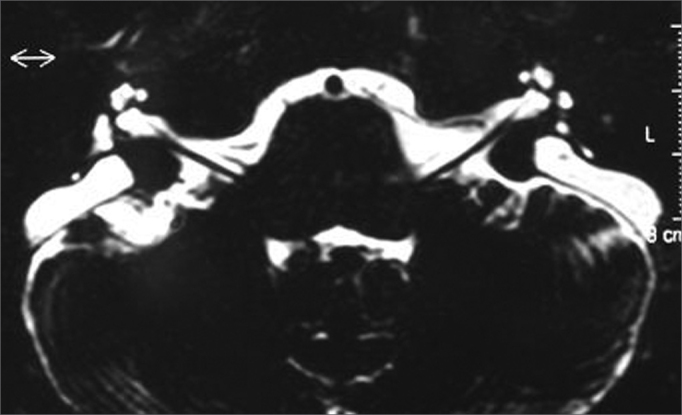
Left side enlarged vestibular aqueduct - Temporal bone MRI, 3D reconstruction.
